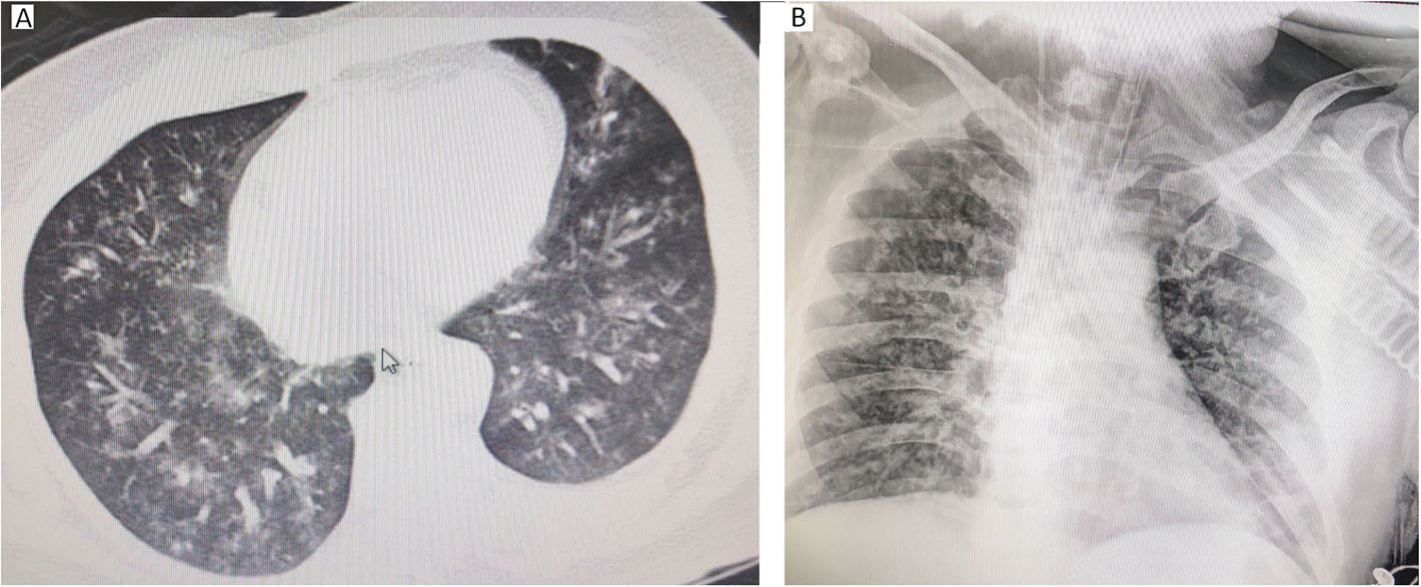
a Image of Chest CT scan and b Chest radiograph taken at admission
